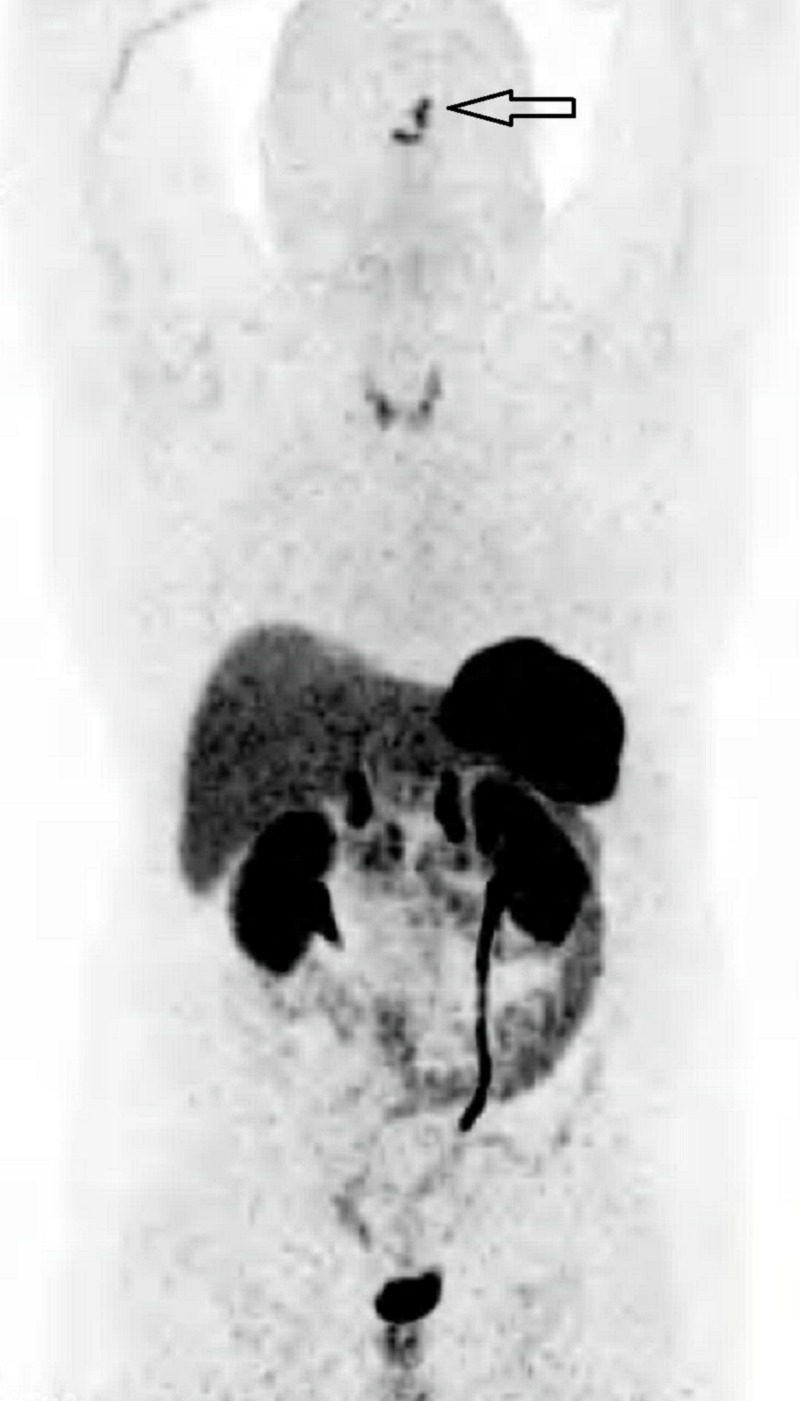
Whole Body FDG-PET/CT Evidence of a small lesion in the left frontal sinus with intense FDG uptake compatible with the recurrence of a bone lesion FDG-PET/CT: 18F-fluorodeoxyglucose positron emission tomography-computed tomography
